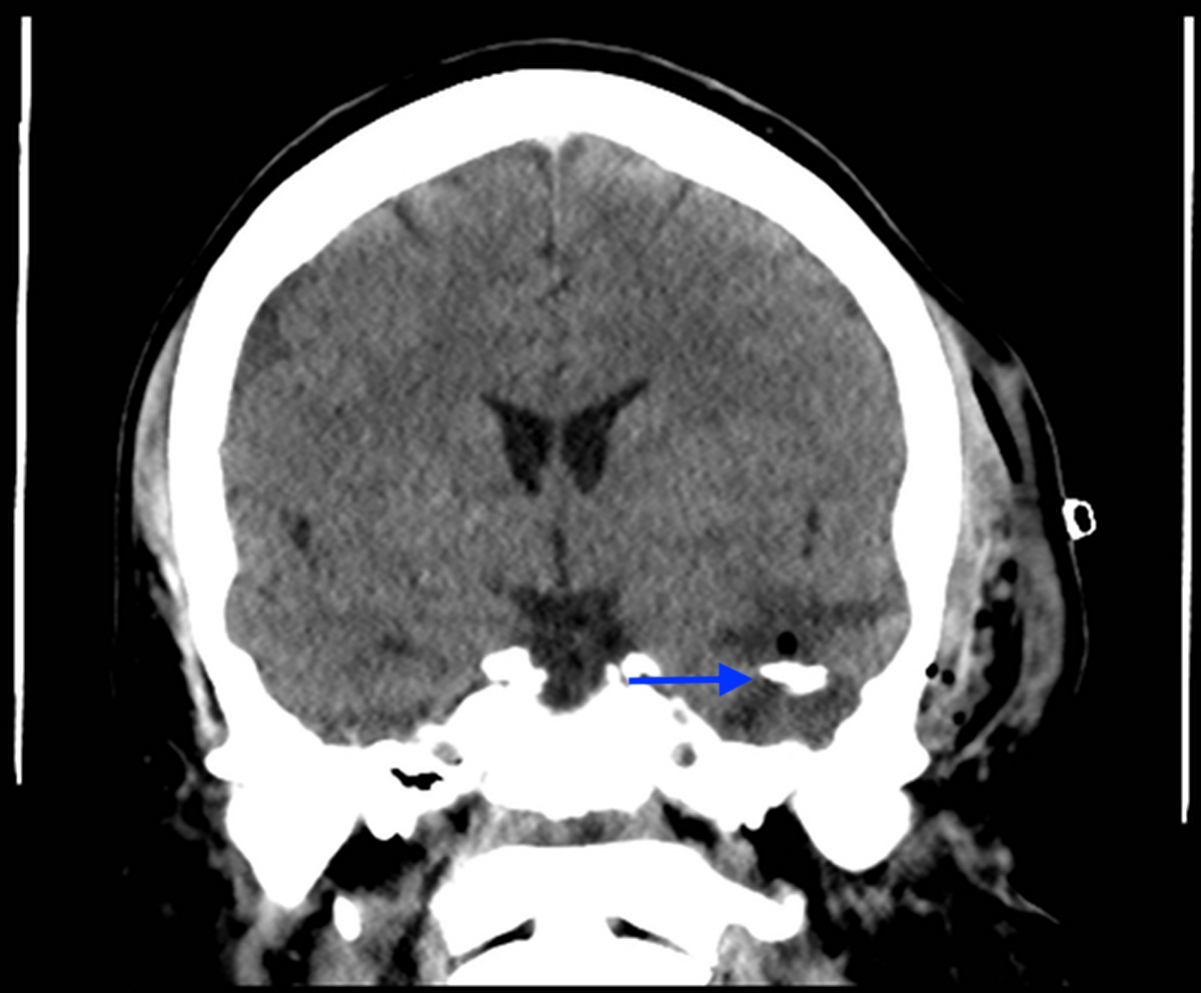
Postoperative unenhanced coronal CT of the head: shunt tubing (blue arrow) sitting in previous cystic cavity, which is now collapsed around the tube. CT - computed tomography
